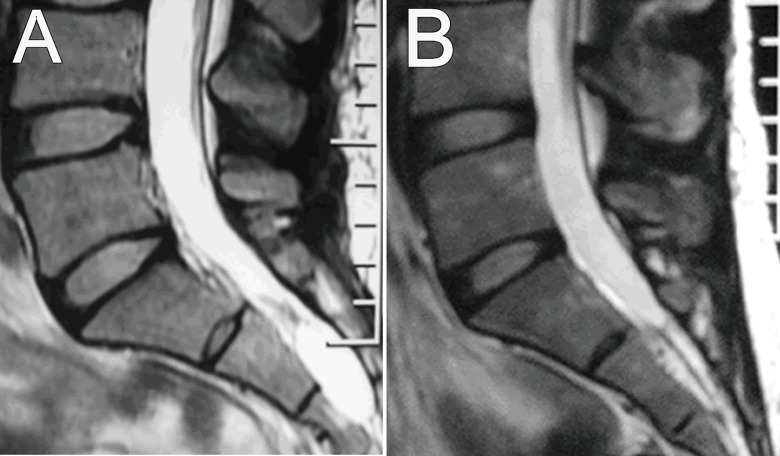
MRI study of cyst disappearance. A. Sagittal T2WI MRI shows cysts before fibrin gel therapy. B. Sagittal T2WI MRI obtained 24 months after operation shows that the cysts disappeared completely.
